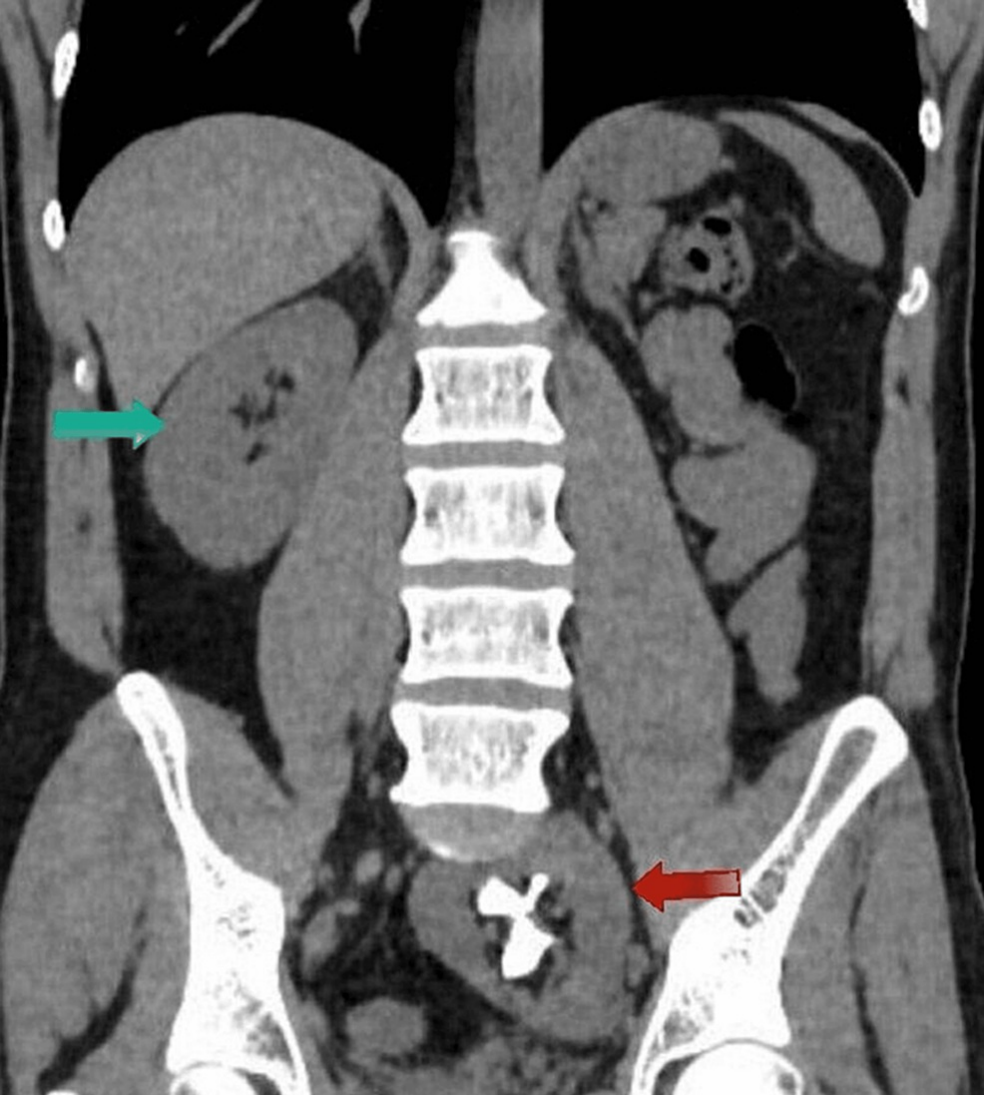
Coronal view CT abdomen showing left pelvic kidney with staghorn stone (red arrow) and normally located right kidney (green arrow)
